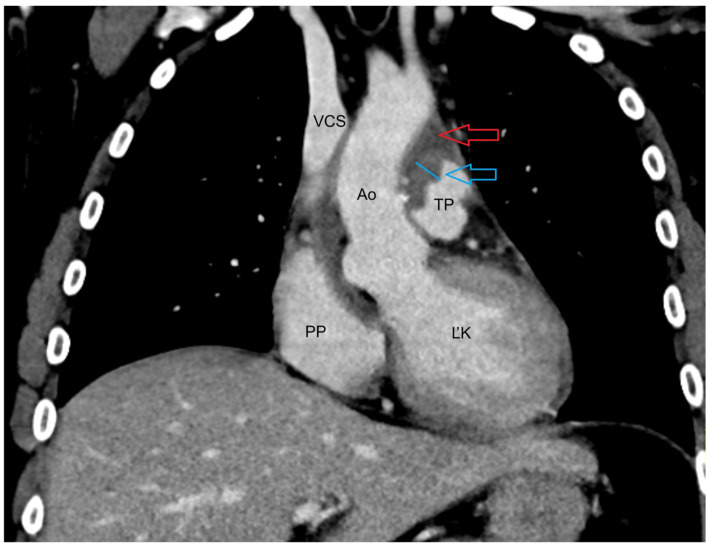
CT of the upper chest venous phase transverse plane: Blue arrow—fluid collection paraaortically to retrosternal with saturating wall, Red arrow—small air collection retrosternal in the fluid collection, Ao—aorta, TP—pulmonary trunk, VCS—superior vena cava, PP—right atrium, ĽK—left ventricule.
